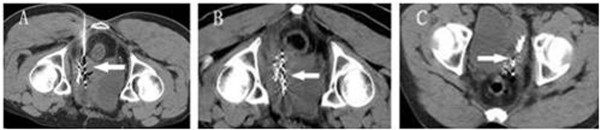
Locally recurrent rectal cancer with ^125^I seeds implant under CT guidance. (a) CT scan shows that ^125^I seeds are implanted into the tumor via 18G implantation needles (arrow). (b) CT scan shows the distribution of ^125^I seeds post implantation (arrow). (c) 2 month follow-up. CT scan shows recurrent tumor partially decreased and ^125^I seeds gathered together (arrow).
